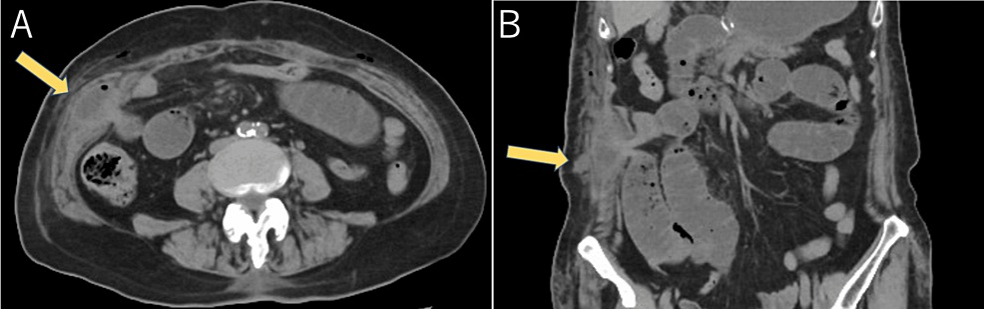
Postoperative axial (A) and coronal (B) CT images in Case 2 The images showed the small intestinal incarceration in the right abdomen (yellow arrows).
